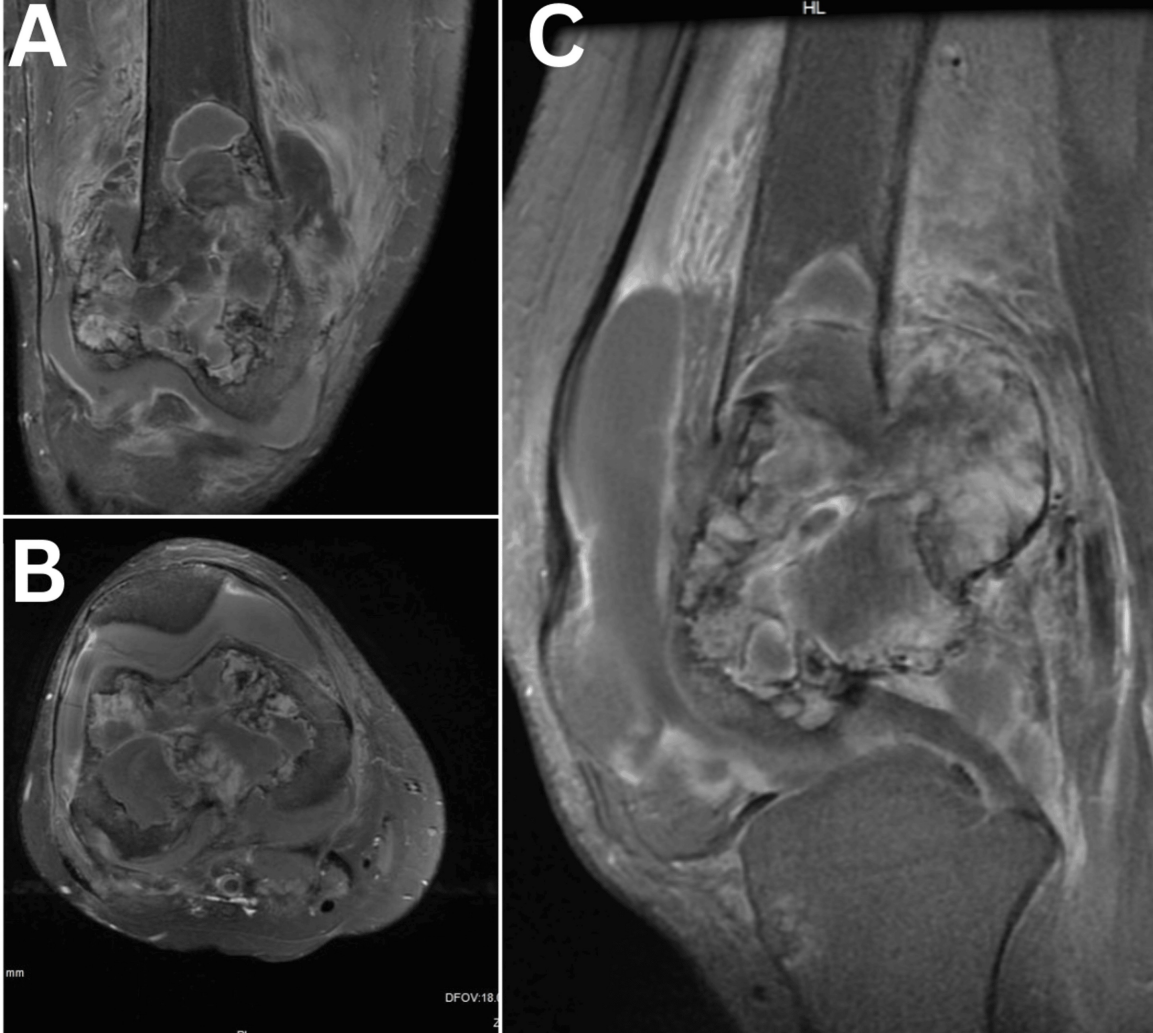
Post-contrast FS T1-weighted images in (A) the coronal, (B) axial, and (C) sagittal planes demonstrate a lesion with heterogeneous enhancement, predominantly along the periphery.
